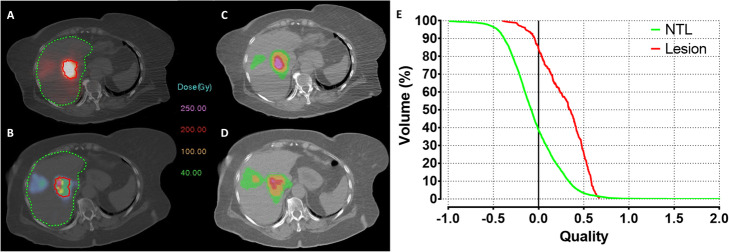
Axial images of a patient with liver mCRC and corresponding QVH. a Axial ^90^Y-PET/CT image on which target lesion and NTL volumes have been projected, showing a high ^90^Y-microsphere uptake within the lesion. b Corresponding axial ^99m^Tc-MAA SPECT/CT. c, d Post-treatment and predictive absorbed dose matrices. e Representation of QVH for both NTL and lesion with a QF of 0.24 and 0.32, respectively
